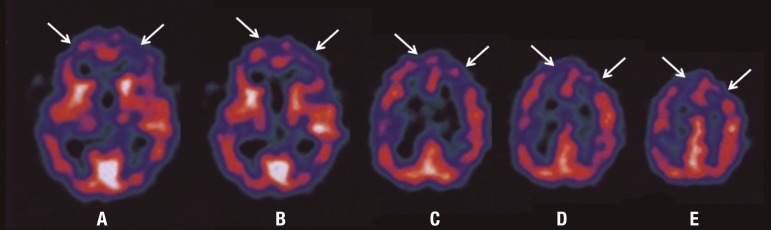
SPECT – axial. A to E: arrows indicating frontal hypoperfusion areas.
